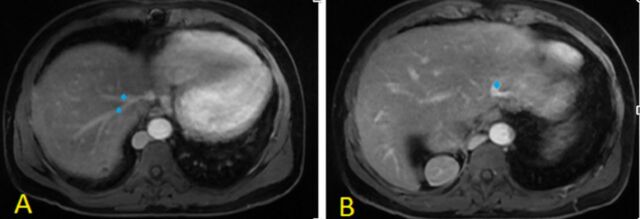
(A, B) Postcontrast axial magnetic resonance imaging shows the hepatic veins (blue diamonds), draining directly into the right atrium.
